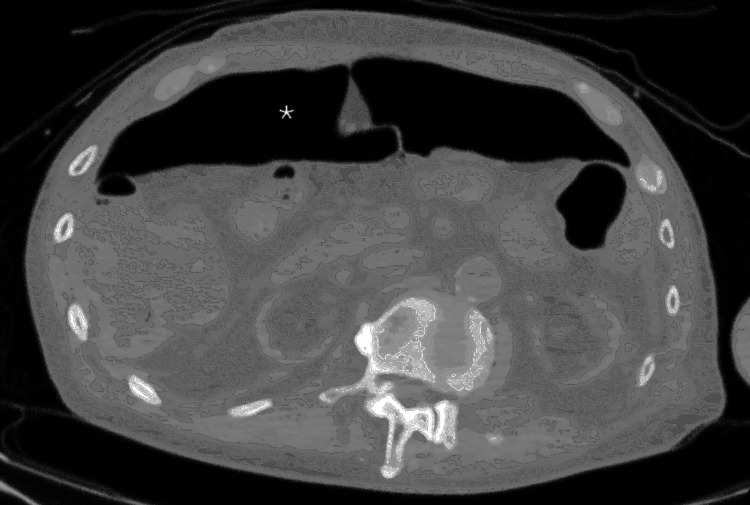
CT after endoscopic intervention. Axial plain CT demonstrates pneumoperitoneum, as indicated by the asterisk, without associated intra-abdominal fluid collection.
